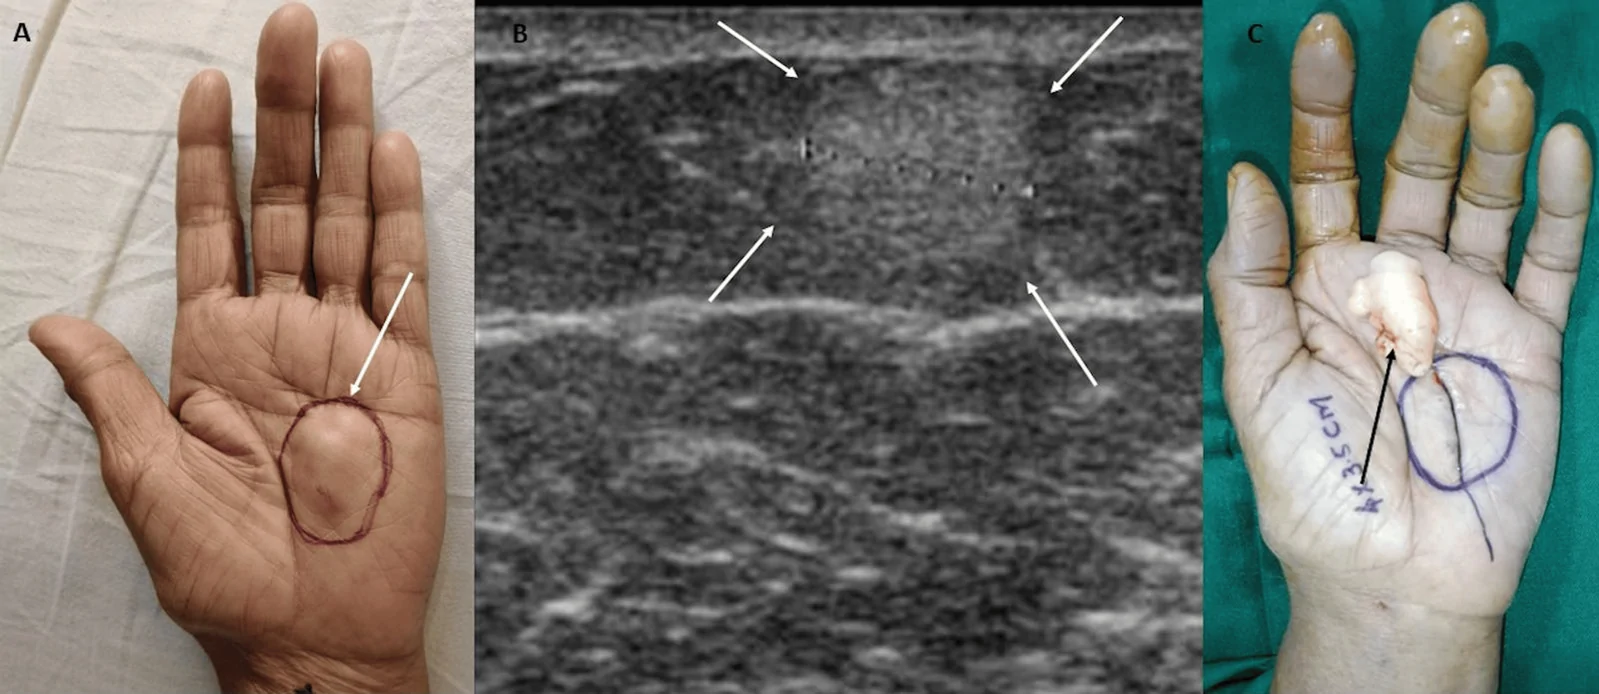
Clinical, radiological, and intraoperative findings of mid palmar swelling (A) Lobulated swelling in the mid-palmar region observed clinically. (B) The ultrasound image shows a well-defined, hypo- to isoechoic, heterogeneous swelling in the subcutaneous tissue. (C) Intraoperative excision demonstrating the gross appearance of the tumor, and the arrow shows the origin from a nerve fiber
